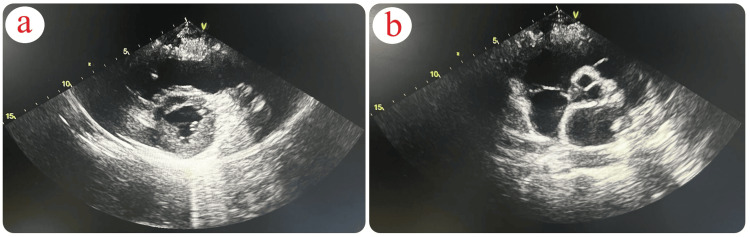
Normal Echocardiogram on ICU Day 4 (a) Transthoracic echocardiogram (parasternal short-axis view) showing the restoration of normal right ventricular size. (b) Transthoracic echocardiogram (parasternal short-axis view, at aortic valve level) showing the resolution of the thrombus.
